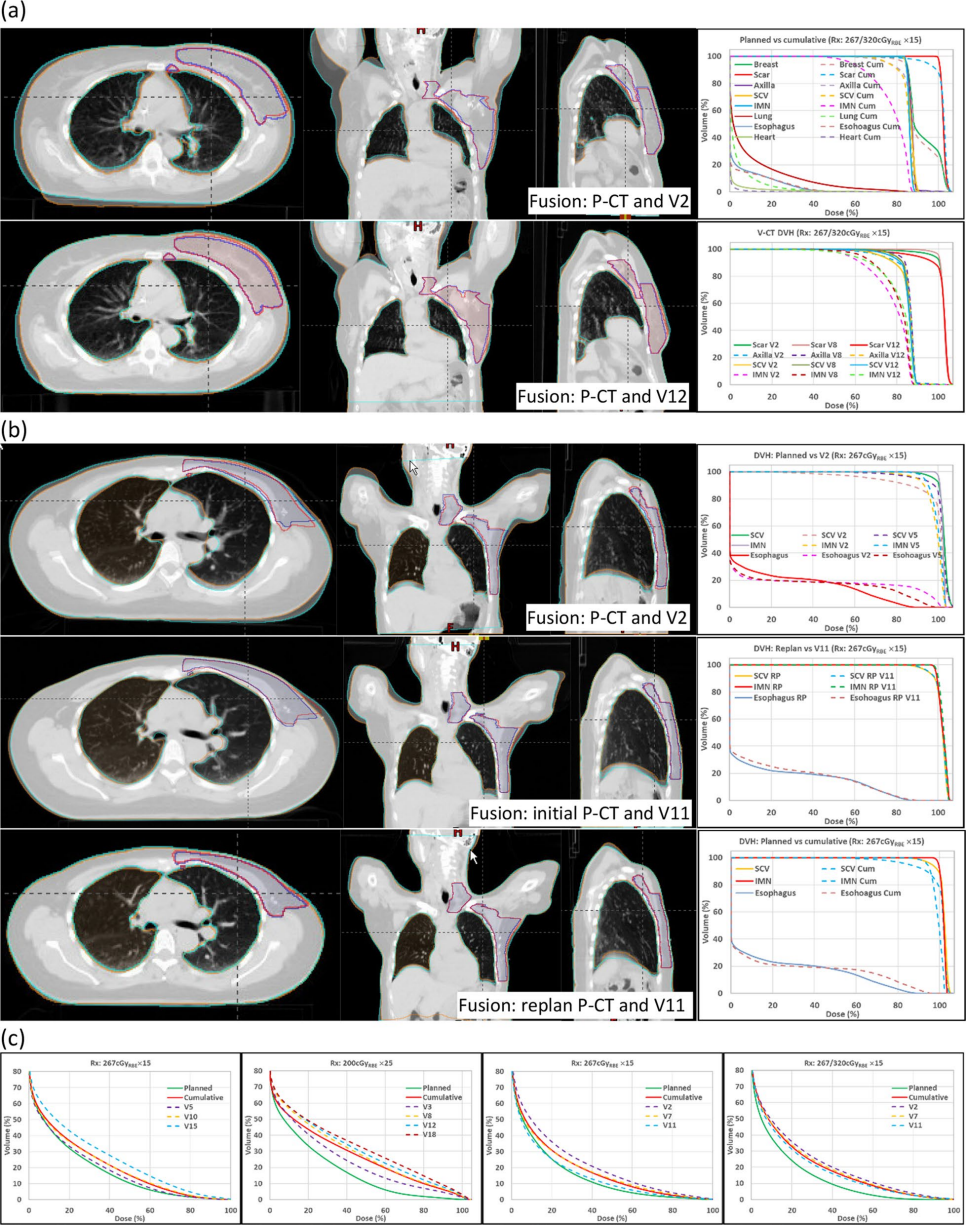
Maskless patients with cumulative dose CTV and OAR LDDs. a A patient with cumulative dose boost, SCV, and IMN D95% LDDs, b A patient with cumulative dose SCV and IMN D95% LDDs, and c Four maskless patients with cumulative dose lung LDDs. P-CT, planning computed tomography; V-CT, verification computed tomography; Vx, V-CT at fraction x; Rx: prescription; Gy_RBE_, Relative-Biological-Effectiveness dose in Gray; cum, cumulative; SCV, supraclavicular node; IMN, internal mammary nodes; RP, replan; CTV, clinical target volume; OAR, organ at risk
